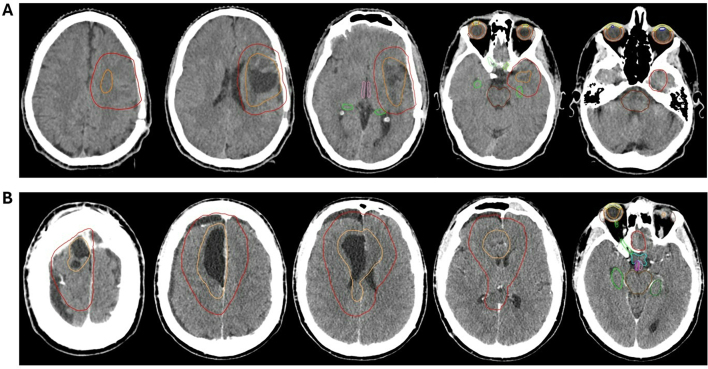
Figure displaying axial dose planning CT images including consensus delineations.
